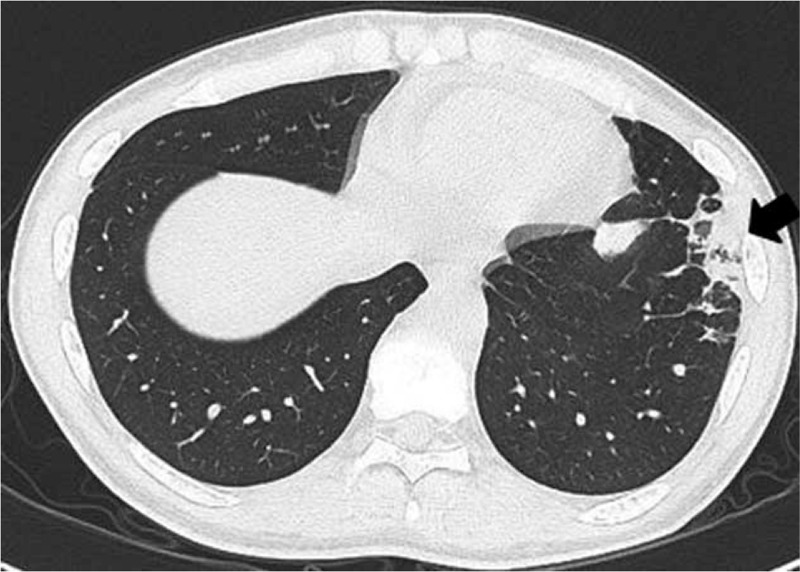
CT showed subpleural wedge-shaped consolidation in the area that was perfused by the obstructed pulmonary artery (arrow).
